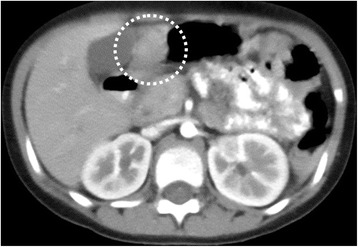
The abdominal contrast-enhanced CT with oral contrast detected a slightly enhanced solid mass at the antrum with no swallowed contrast inside (*dotted circle*)
